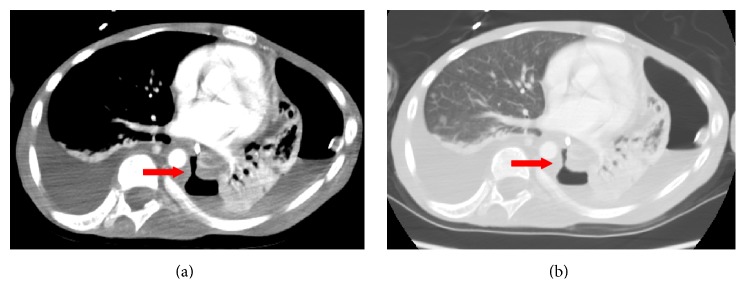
Chest CT with wide window width: (a) mediastinal window, (b) lung window showing esophageal tear and communication with pleura (red arrow).
